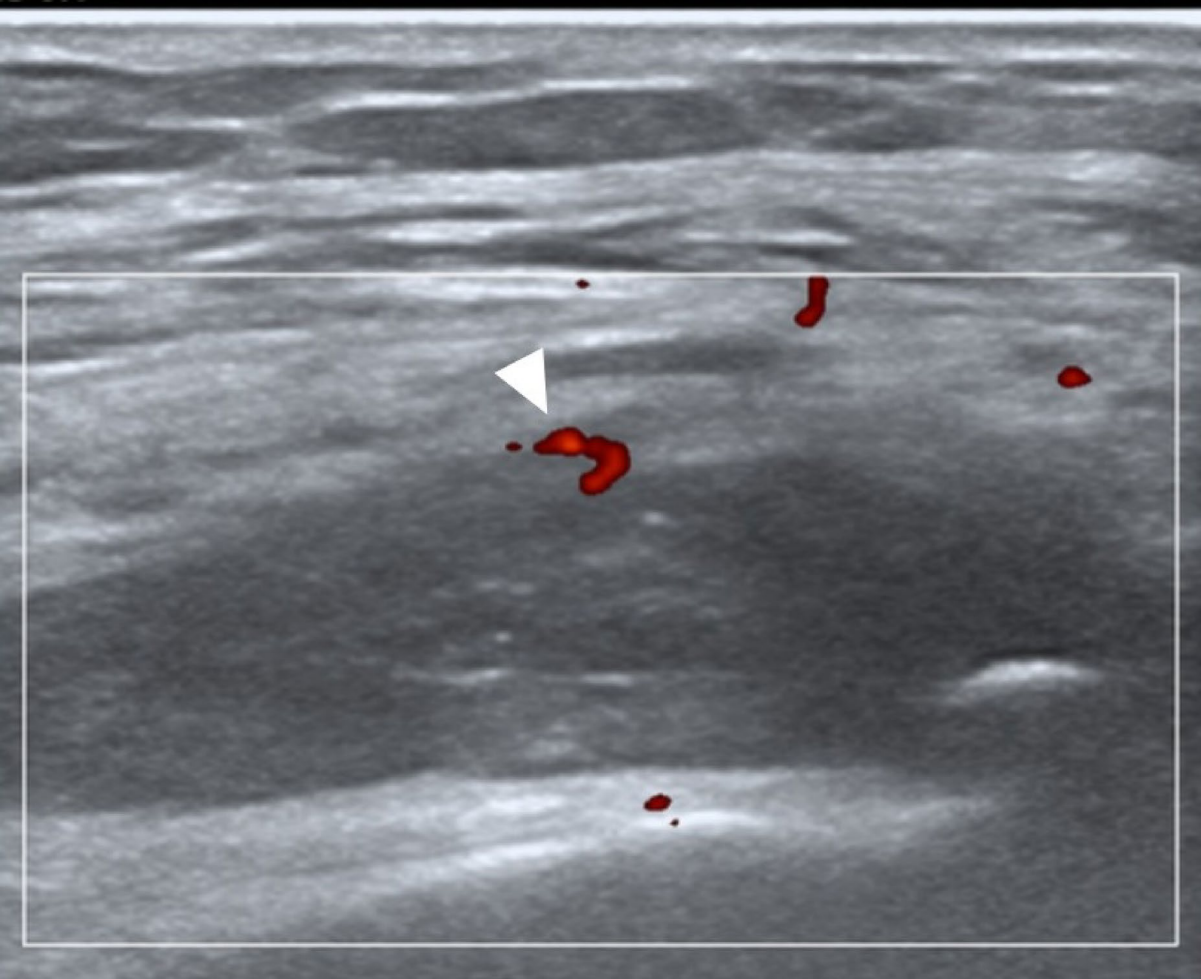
Power Doppler (PD) sonogram. Example of ultrasound imaging showing grade 1 (mild) PD joint inflammation at the supra-patellar recess of the knee (white arrowhead pointing towards PD vascularity) in a patient with rheumatoid arthritis.
